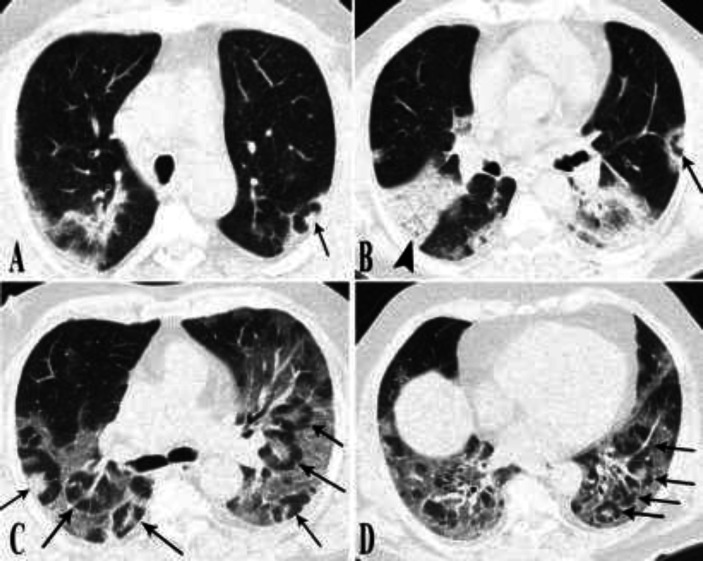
Chest CT scans of cases 5-6.
